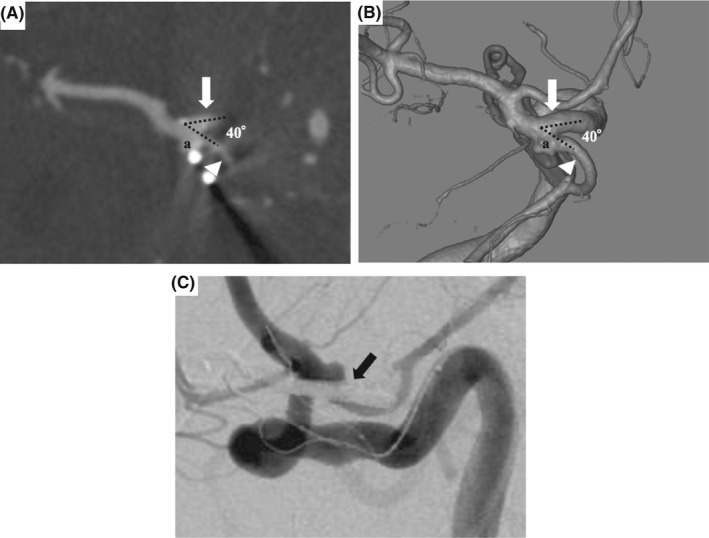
Axial computed tomography angiography source images (A) and 3‐dimensional digital subtraction angiography (image from directly above) (B) demonstrating a 2.7‐mm broad‐necked posterior communicating artery (PcomA) aneurysm residuum (a) just proximal to the clip incorporating an acute angled fetal variant posterior cerebral artery. These images show an acute angle (40°) subtended by the PcomA (white arrowhead) and proximal internal carotid artery (white arrow). The orifice of the PcomA is not well visualized on right internal carotid artery angiography (C) due to the existing clip
